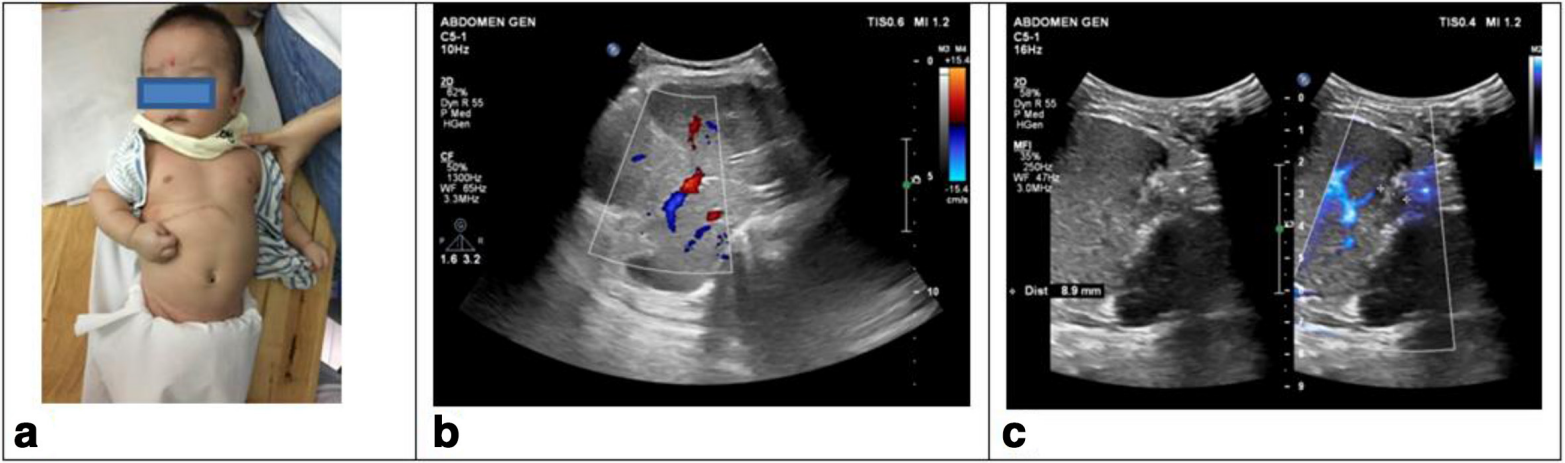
Pictures of the paediatric patient and abdominal ultrasound after the surgery.
